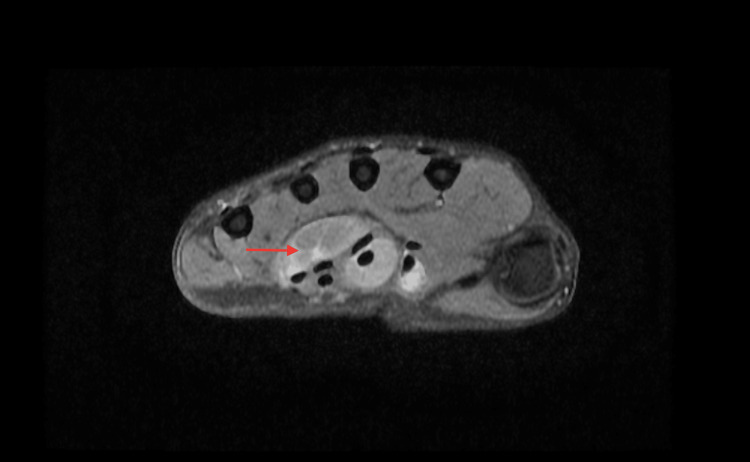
MRI of the left hand in axial view showing diffuse thickening of the synovium and non-enhancing nodules in the tendon sheath of the flexor digitorum superficialis
